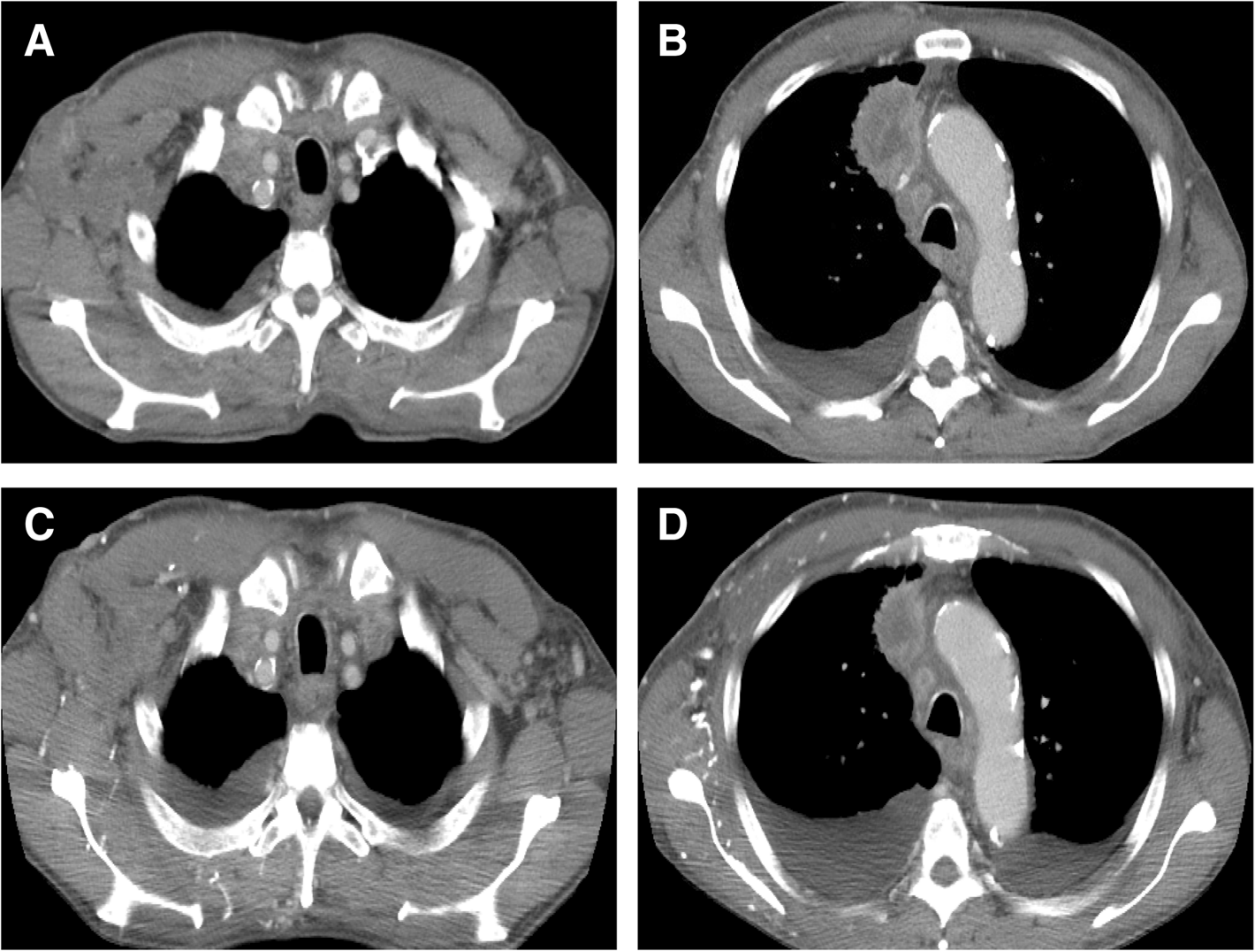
Chest computed tomography analysis determines the baseline before pembrolizumab therapy. A tumor approximately 43 mm in diameter in the right upper lobe, right axial and mediastinal lymph node metastases, and pleural effusion were observed (a, b). After palliative radiotherapy, the size of the right axial lymph node metastasis was decreased (c, d)
